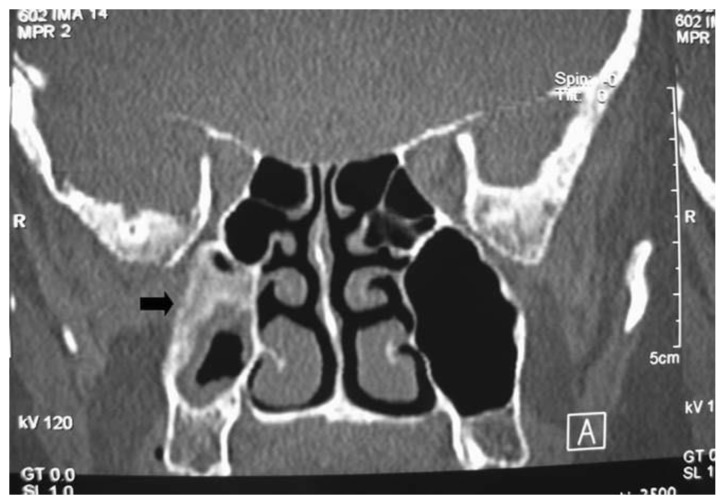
Coronal image of computed tomography showing a recurrent sinonasal inverted papilloma pedicled on the posterior maxillary sinus wall. There is significant hyperostosis at the origin of the lesion.
